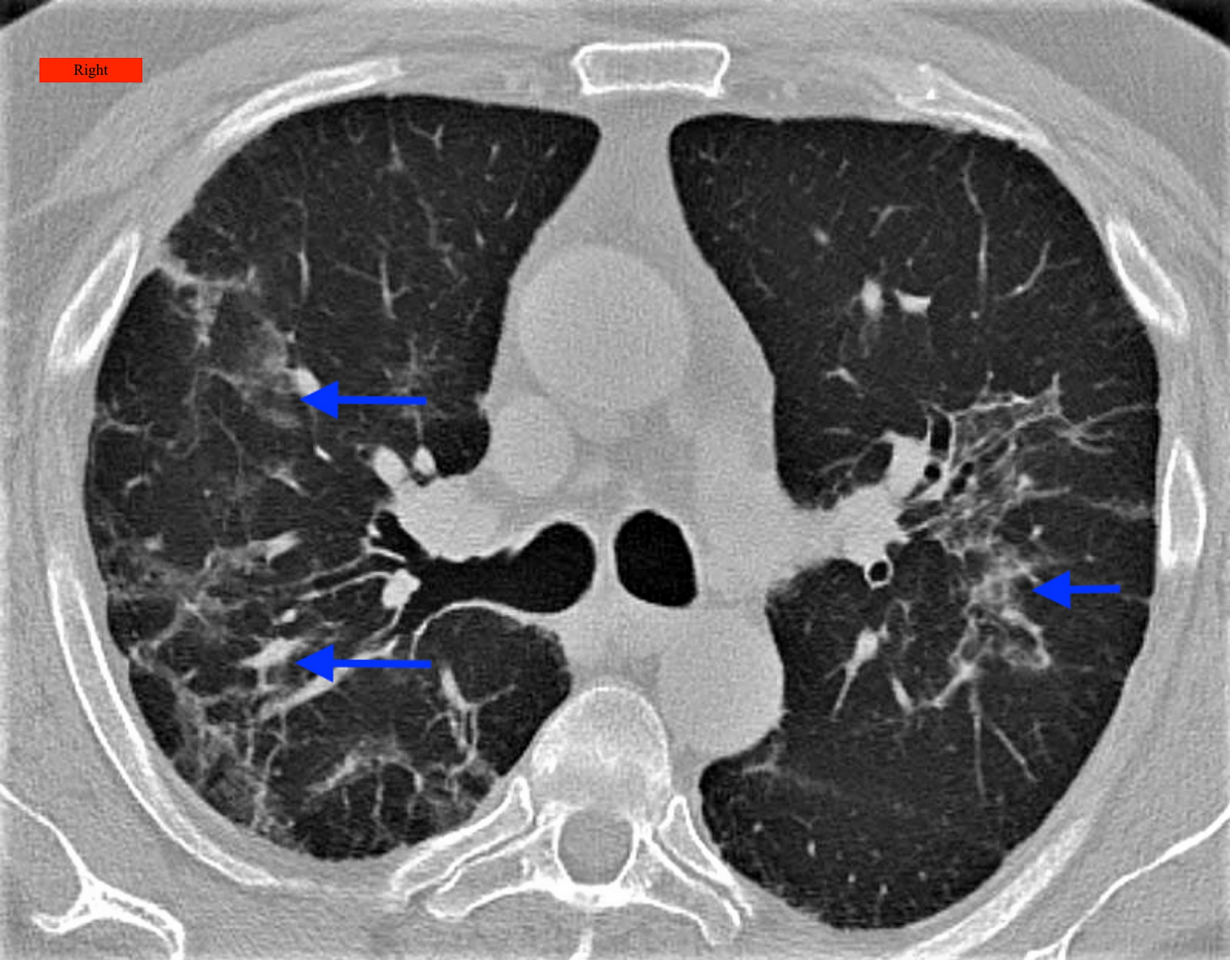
A chest CT showed areas of bilateral diffuse ground-glass opacities, interstitial thickening, and bronchial dilatation (arrows) Right: Indicates the right side of the patient; CT: Computed Tomography
